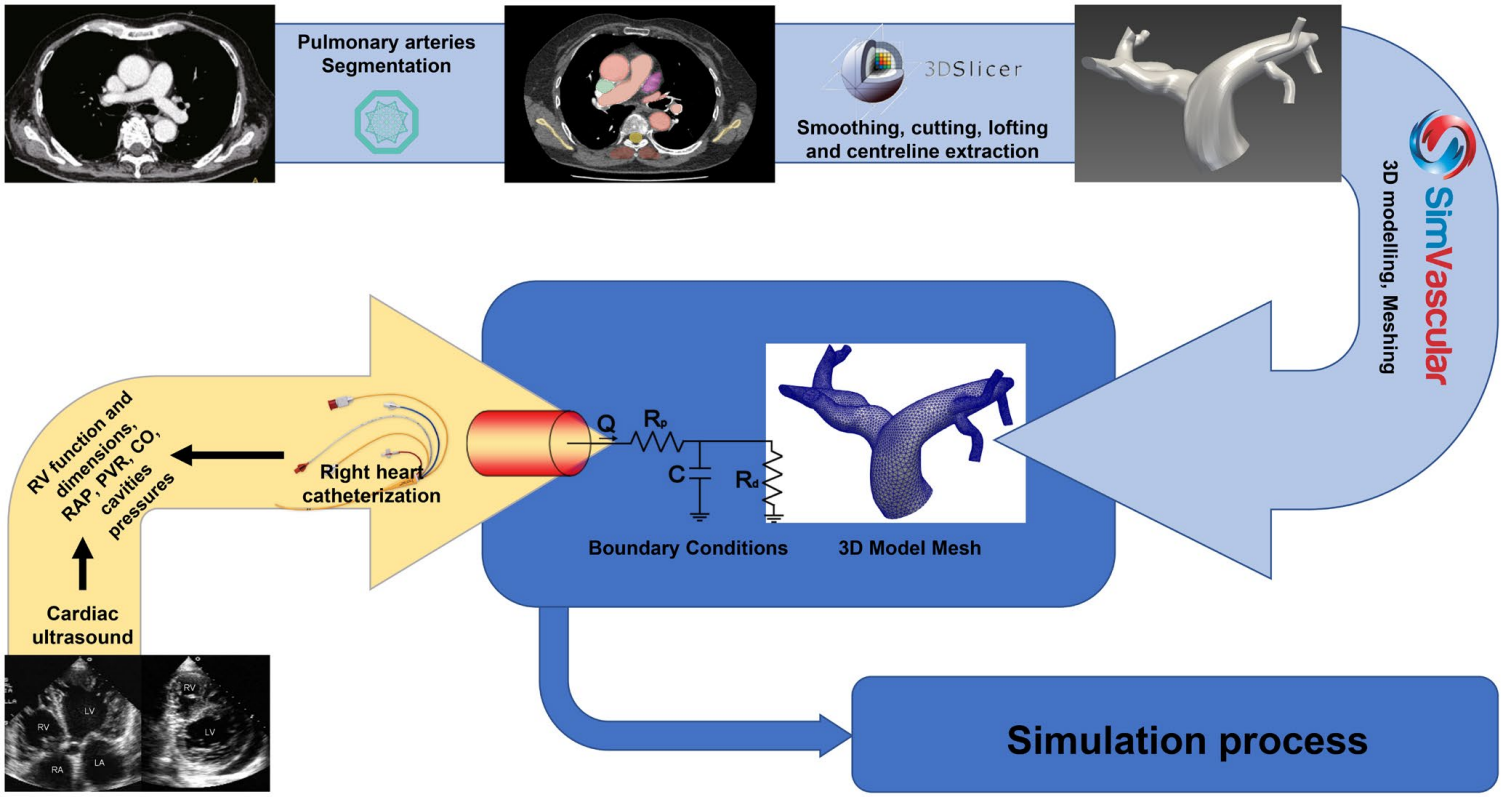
Three‐dimensional pulmonary artery model reconstruction pipeline. Pulmonary artery segmentation, 3D models reconstruction (3D Slicer), and meshing (SimVascular) were combined with patient‐specific boundary conditions derived from cardiac ultrasound and right heart catheterization. CFD, computational fluid dynamics; CO, cardiac output; PAP, pulmonary artery pressure; PCWP, pulmonary capillary wedge pressure; RAP, right atrial pressure; RV, right ventricle.
